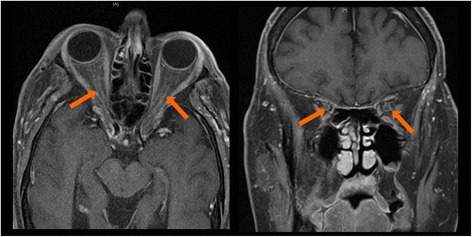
MRI orbits after onset of right eye vision loss, demonstrating subtle circumferential perineural enhancement of bilateral optic nerves, demonstrated on axial and coronal views consistent with optic nerve inflammation (highlighted by the arrows)
